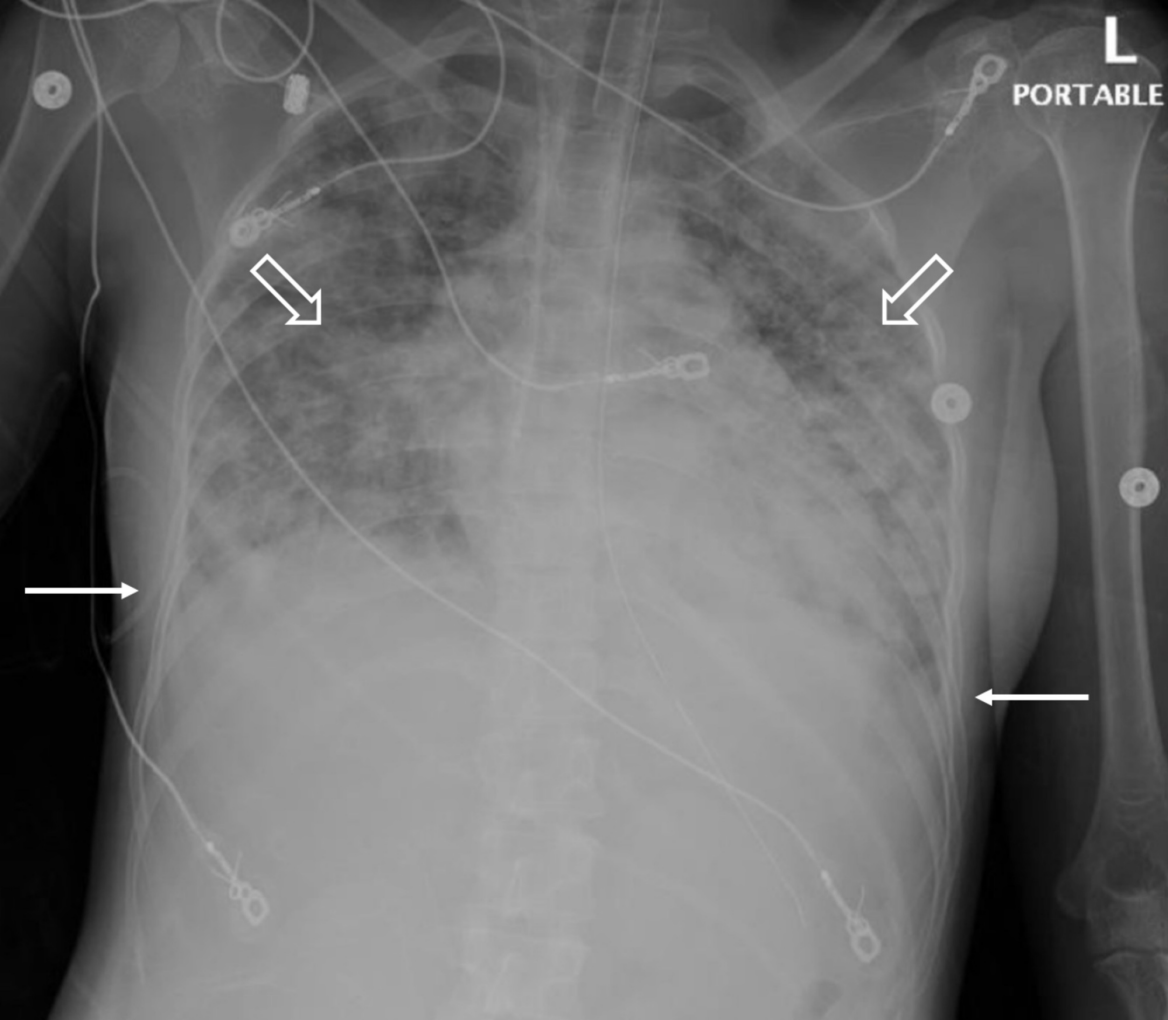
Chest X-ray on hospital day one showing diffuse pulmonary edema (blank arrows), and bilateral airspace disease with bilateral pleural effusions (solid arrows).
